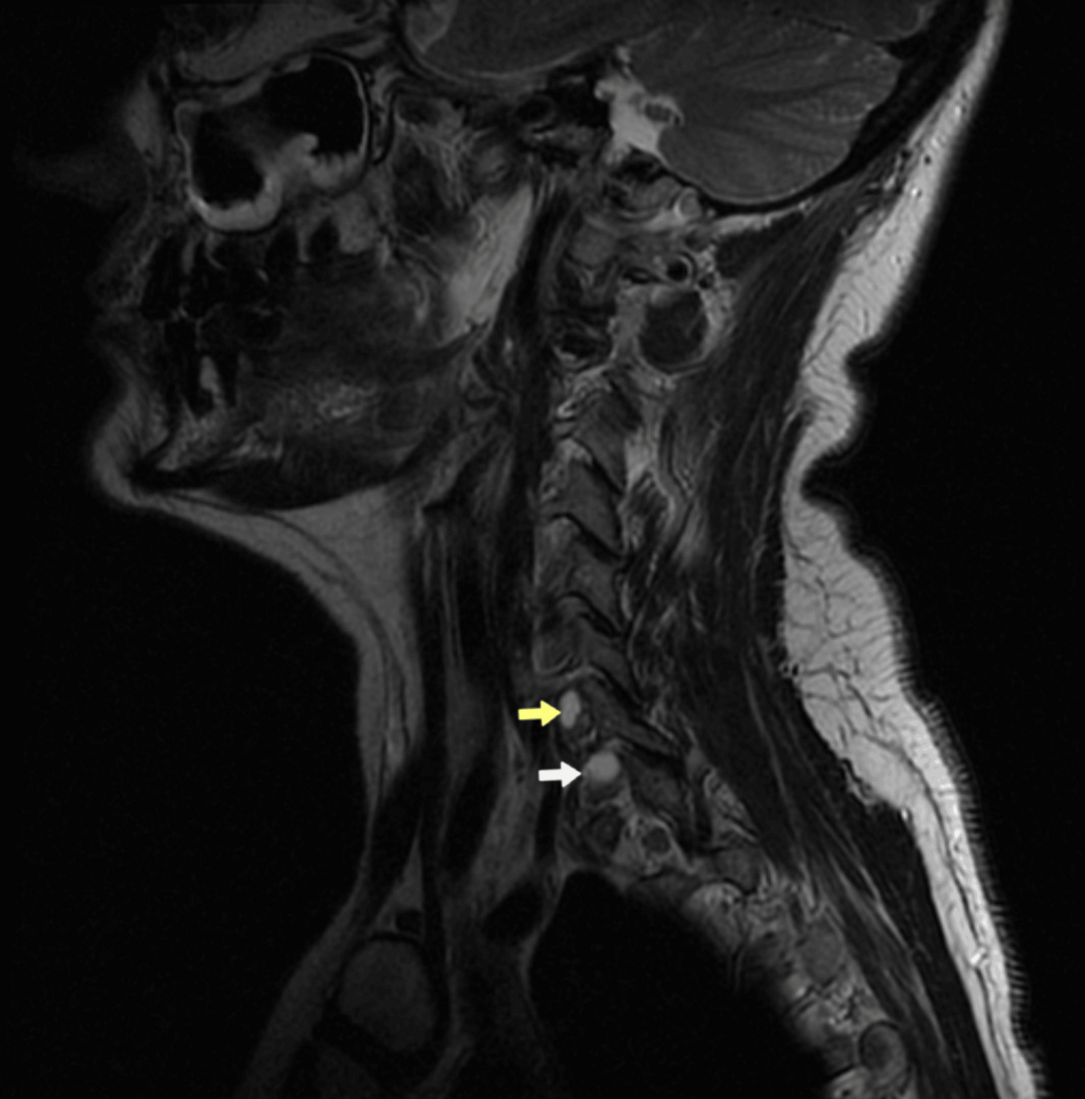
Magnetic resonance image of the cervical spine. Sagittal T2-weighted image showing two Tarlov cysts in the right foramen at levels C6-C7 (yellow arrow) and C7-T1 (white arrow), resulting in the compression of the C7 and C8 nerve roots, respectively, explaining the presence of neurological symptoms in two distinct dermatomes.
